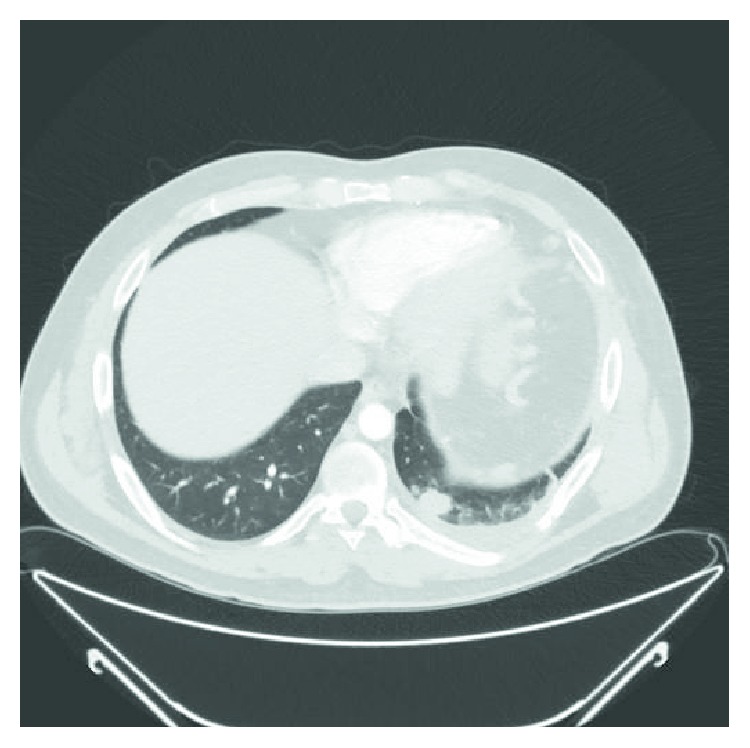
Computed tomography of the chest from May 2012 showing a left lower lobe residual nodular density.
